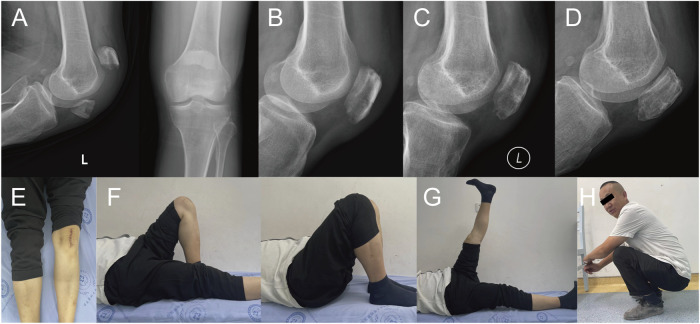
A 51-year-old male with a left TPF from a fall. X-rays: (A) Before the fracture. (B) The first day after surgery. (C) Three months after surgery. (D) Twelve months after surgery. (E) External observation; (F–H) Functional reexamination photos 1 year after surgery.
